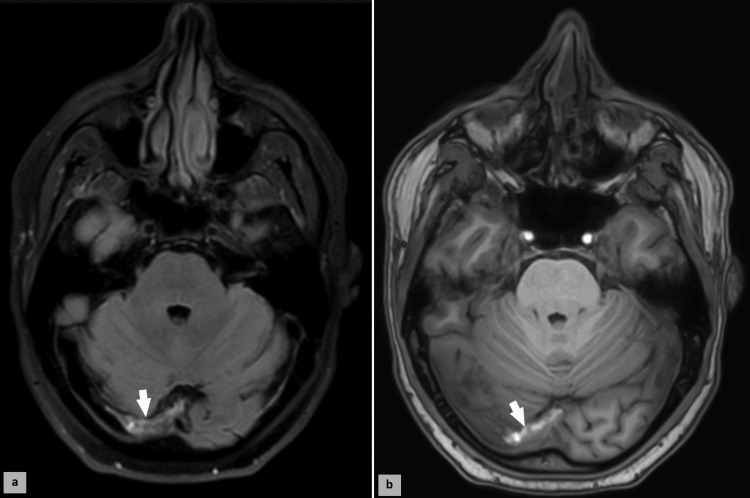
MRI of the brain (axial views): (a) T1-weighted image demonstrating left lateral venous thrombosis in the posterior fossa (arrow), and (b) T1-weighted contrast-enhanced image depicting the corresponding thrombus with hyperintense signal (arrow).
